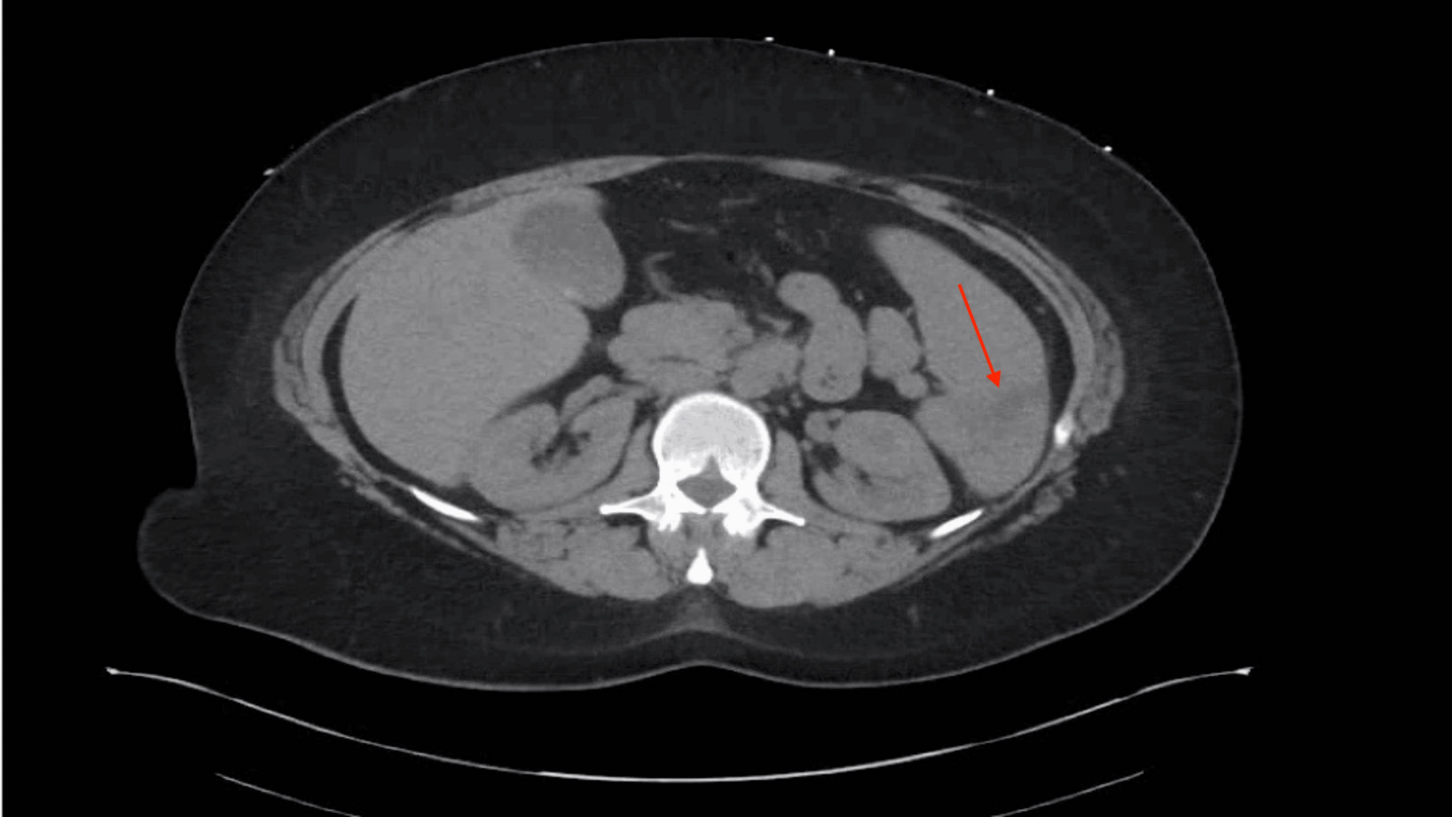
A repeat CT scan of the abdomen showing a resolving splenic infarct (red arrow).
